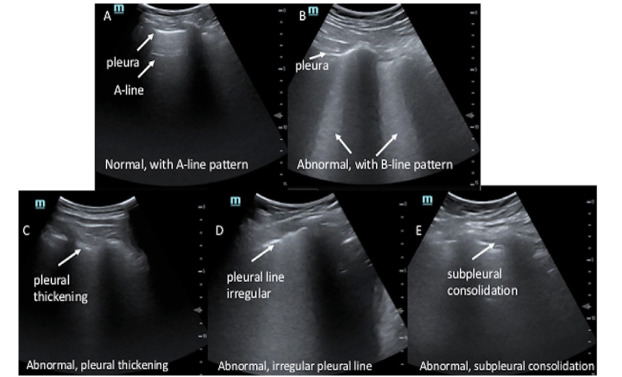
Lung ultrasounds. (A) Normal lung ultrasound. A-lines are horizontal lines that can be seen in the absence of pathology. (B) Abnormal lung ultrasound. The pleura is noted at the top of the lung. This is an example of coalescing B-lines shown as what appear to be headlights coming down from the pleura. (C) Abnormal lung ultrasound. Demonstrated is pleural thickening, >3 millimeters by visual estimate was considered abnormal. (D) Abnormal lung ultrasound. Demonstrated is an irregular pleural line seen in viral infections. (E) Abnormal lung ultrasound. Shown is a subpleural consolidation that appears black between the pleura above the pleural line.
